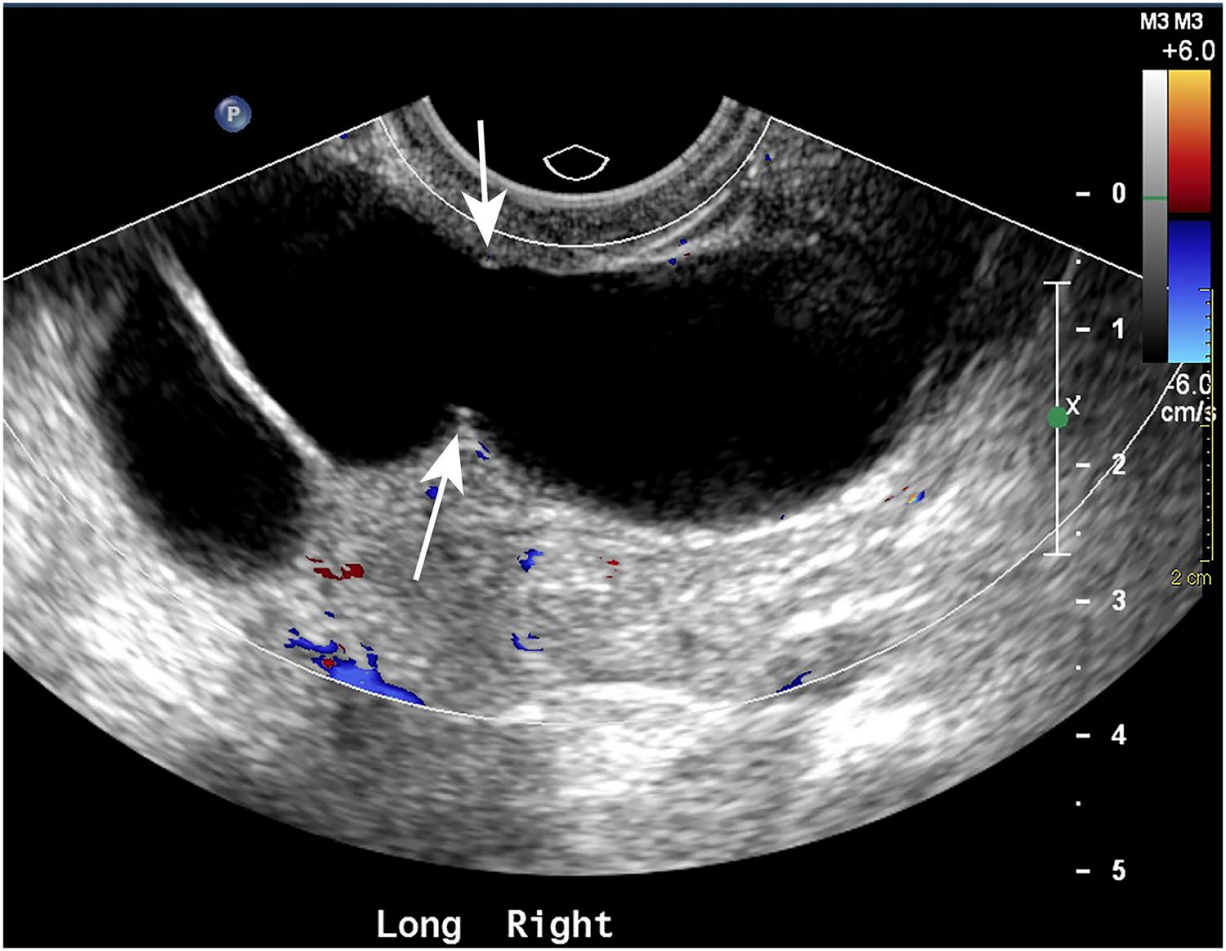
Color Doppler ultrasound image of the right adnexa shows a tubular, avascular, cystic structure with a “waist” (arrows), consistent with a hydrosalpinx
